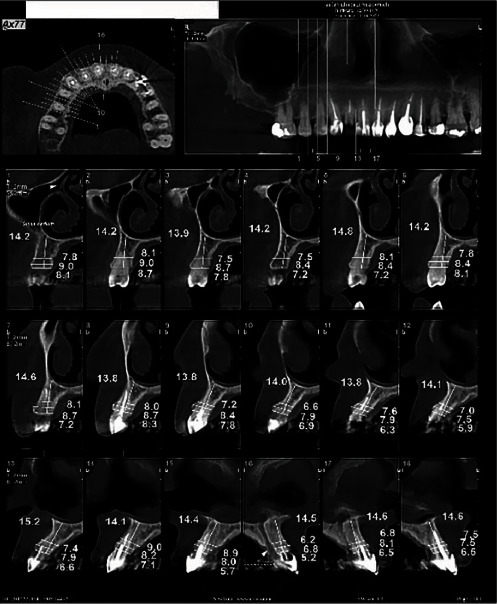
Preoperative CBCT scan of a patient. CBCT, cone-beam computed tomography.
